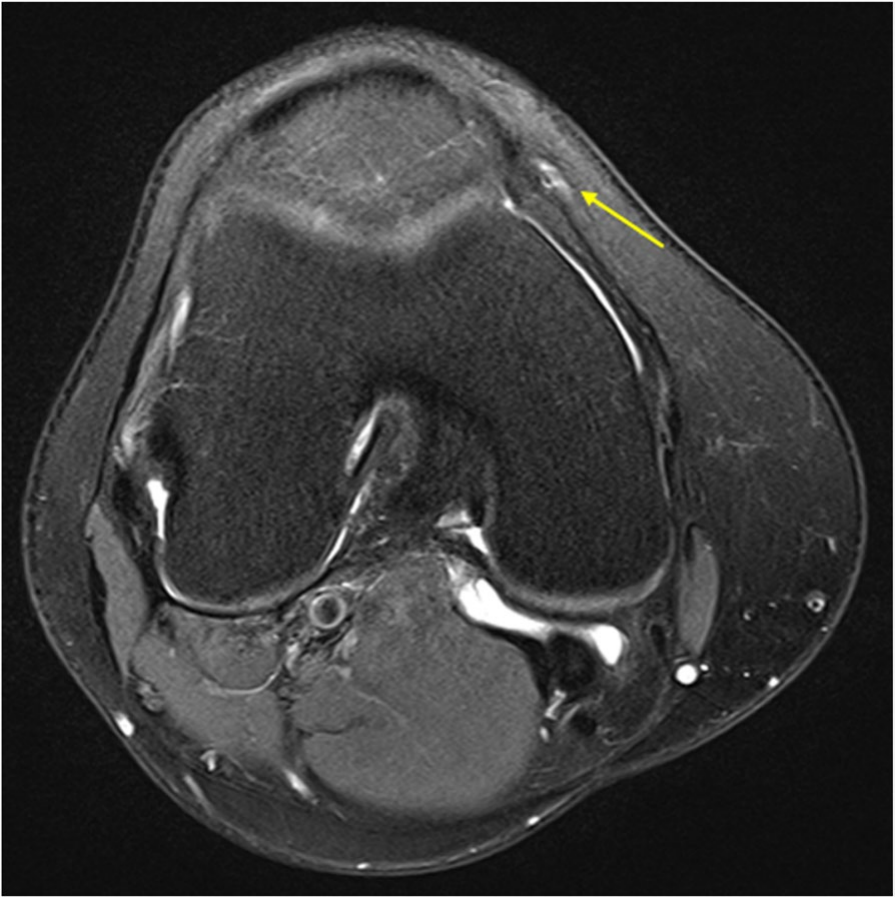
MRI of the left knee. Axial PDFS sequence. Fibrosis of the lower medial retinaculum. The infrapatellar branch of the saphenous nerve is oedematous (yellow arrow)
